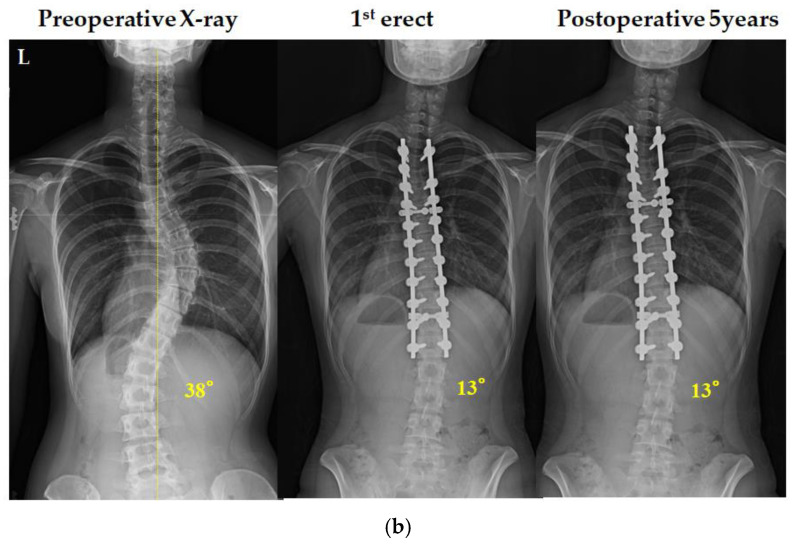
X-rays show a thirteen-year-old girl with Lenke 1C scoliosis (a). The preoperative X-ray shows a thoracolumbar/lumbar Cobb angle of 35°. The postoperative X-ray at 1st erect shows a lumbar Cobb angle of 15° after posterior fusion and correction using the CRT technique. The postoperative X-ray at the final follow-up shows a lumbar Cobb angle of 13°. The X-rays show an eighteen-year-old girl with Lenke 1C scoliosis (b). The preoperative X-ray shows a thoracolumbar/lumbar Cobb angle of 38°. The postoperative X-ray at 1st erect shows a thoracolumbar/lumbar Cobb angle of 13° after posterior fusion and correction using the CRT technique. The yellow dotted line indicates the central sacral vertical line.
